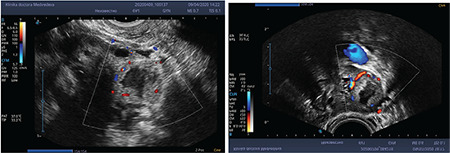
Doppler features of CLC (ring of fire)
